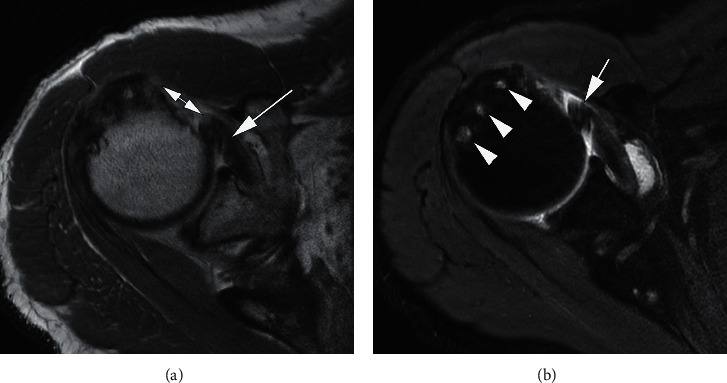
A 49-year-old man with right shoulder pain and inability to extend the right arm after a fall. Axial PD-weighed MRI (a) demonstrates a full-thickness subscapularis tendon tear (long arrow) with medial retraction (short double-headed arrow). Axial T2-weighed FS MRI (b) shows multiple subchondral cysts at the greater tuberosity (arrowheads), which is an associated finding with rotator cuff tears. In addition, there is a medial displacement of the long head of the biceps tendon (arrow).
